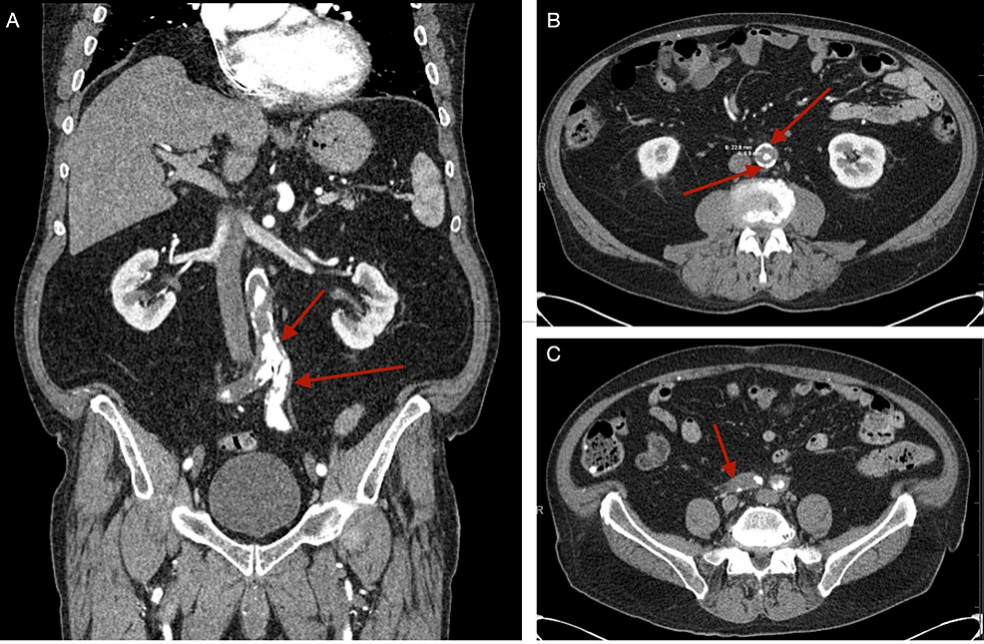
Computed tomography angiogram of the abdominal aorta with bilateral lower extremity runoff: A) Coronal view demonstrating extensive calcific and atherosclerotic disease of aorta extending into right common iliac artery. B) Axial view at level of kidneys demonstrating circumferential atheromatous plaquing noted within abdominal aorta resulting in luminal narrowing up to 70%. C) Axial view below aortic bifurcation demonstrating occlusion of the right common iliac artery.
